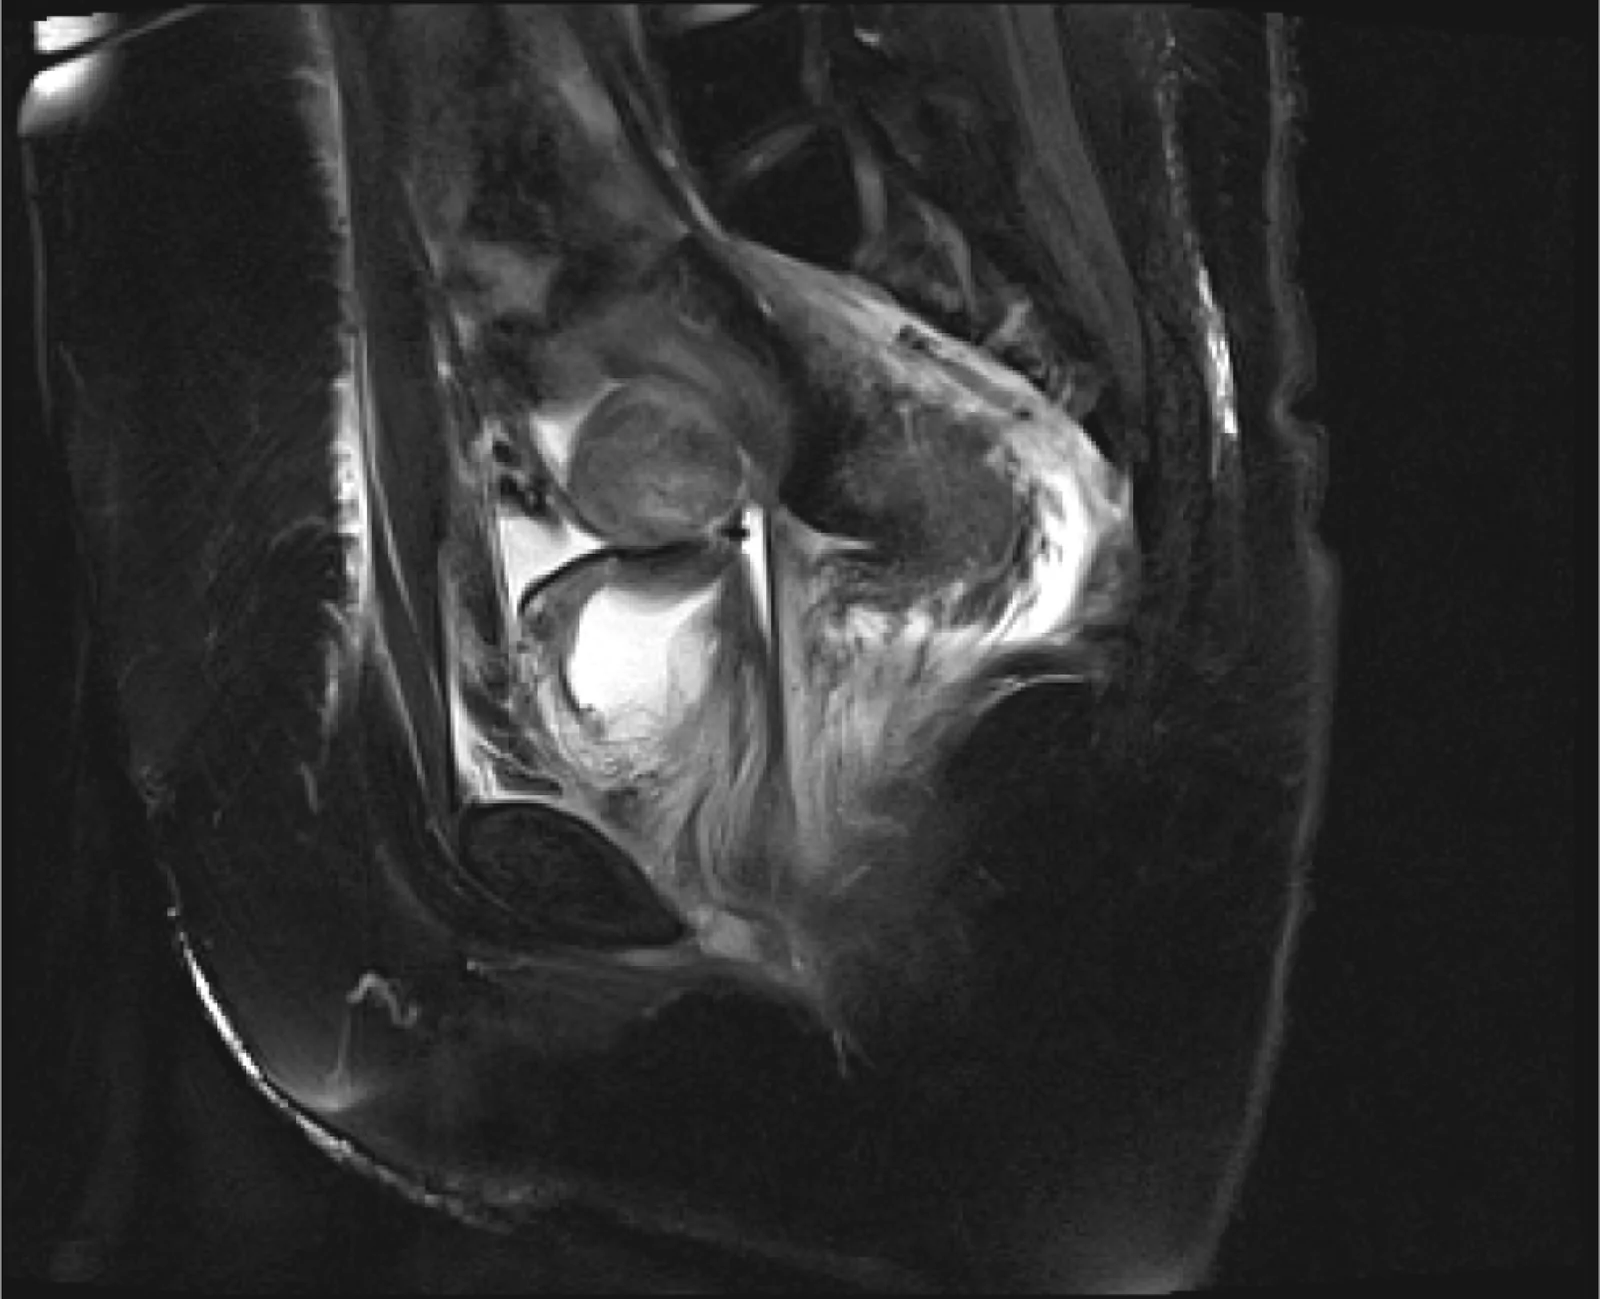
Posttreatment pelvic MRI. Sagittal T2-weighted fat-suppressed sequence acquired after CCRT and bevacizumab therapy demonstrates complete resolution of the previously visualized cervical mass, with no residual abnormal signal or soft tissue thickening, consistent with CR. CCRT = concurrent chemoradiotherapy, CR = complete remission, MRI = magnetic resonance imaging.
